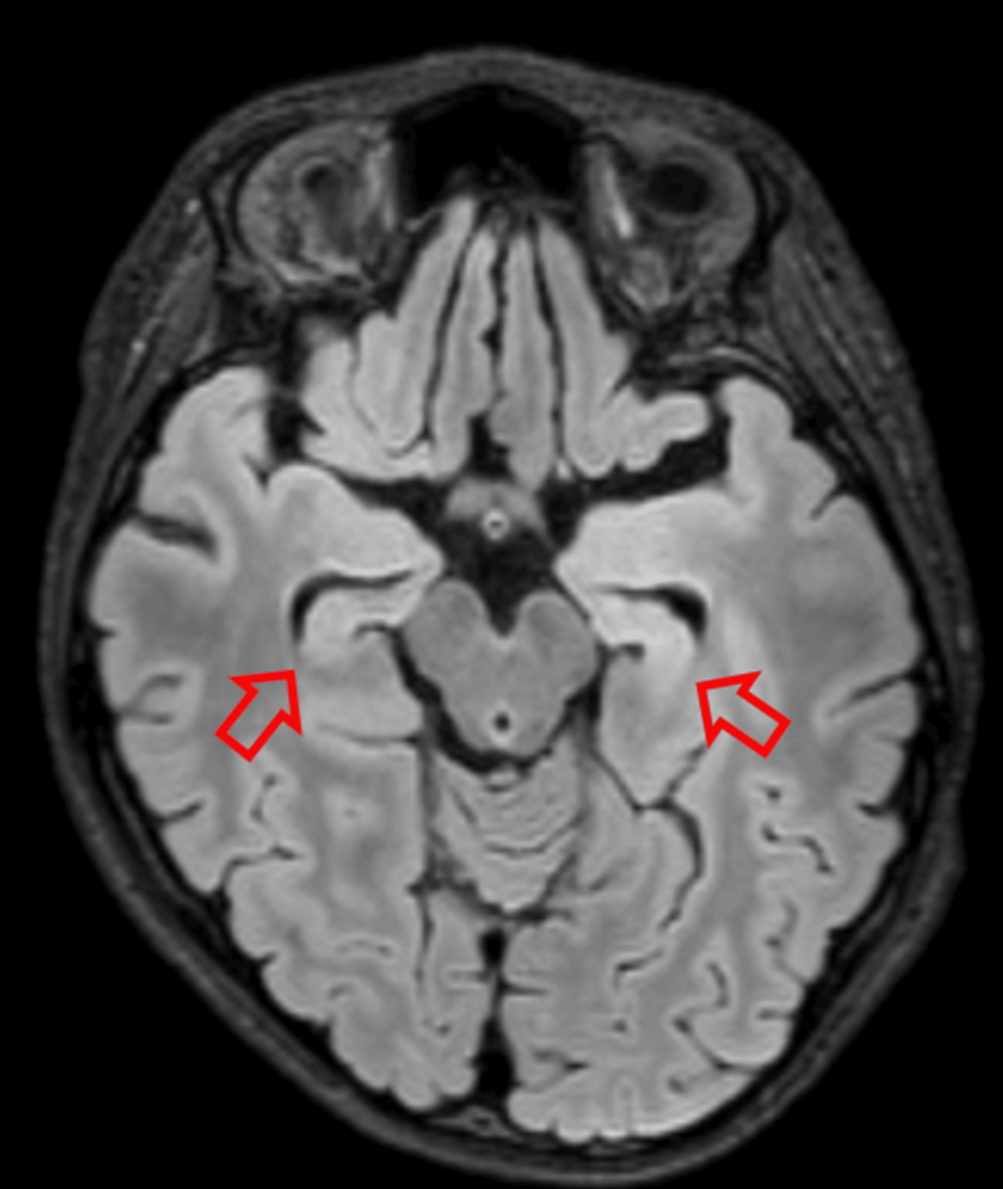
Brain magnetic resonance imaging on day 96 Fluid-attenuated inversion recovery (FLAIR) sequence showing bilateral hippocampal atrophy (red arrows).
